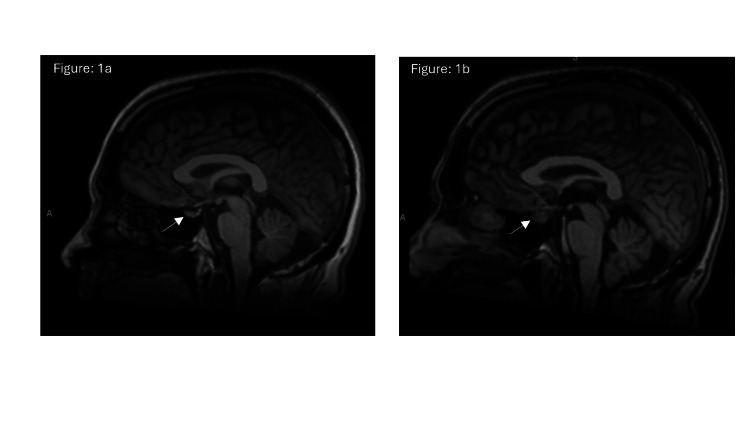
Sagittal T1-weighted post-contrast MRI images of the brain (1a) Represents baseline imaging prior to immune checkpoint inhibitor (ICI) therapy showing a normal-sized pituitary gland. (1b) Shows interval enlargement and heterogeneous contrast enhancement of the pituitary gland consistent with immune-related hypophysitis. Findings supported pituitary inflammation associated with combination ICI therapy.
